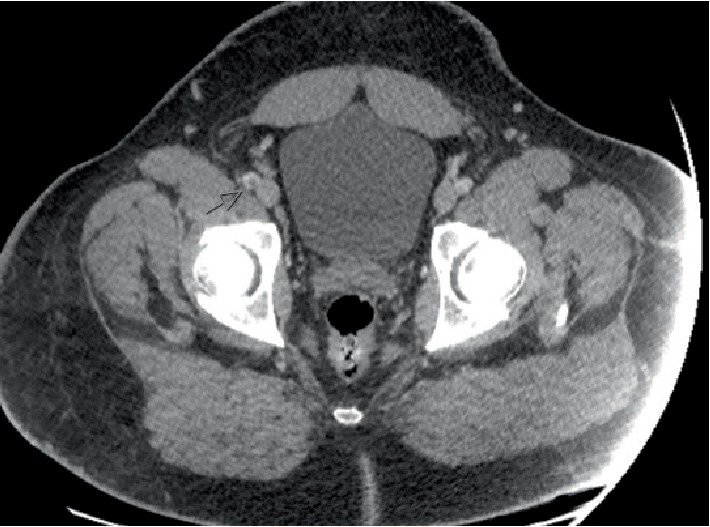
CT angiogram abdominal aorta with right lower extremity runoff shows a nonoccuslive longitudinal filling defect in the distal right external iliac artery and tibioperoneal trunk suggestive of a thrombus. There, however, are Patent common femoral artery, profunda, and SFA. Left lower extremity runoff showed a patent common femoral artery, profunda, popliteal artery and SFA. The abdominal aorta is widely patent and measures 2.3 cm the celiac, 2 cm at the SMA, and 1.7 cm below the renal arteries.
